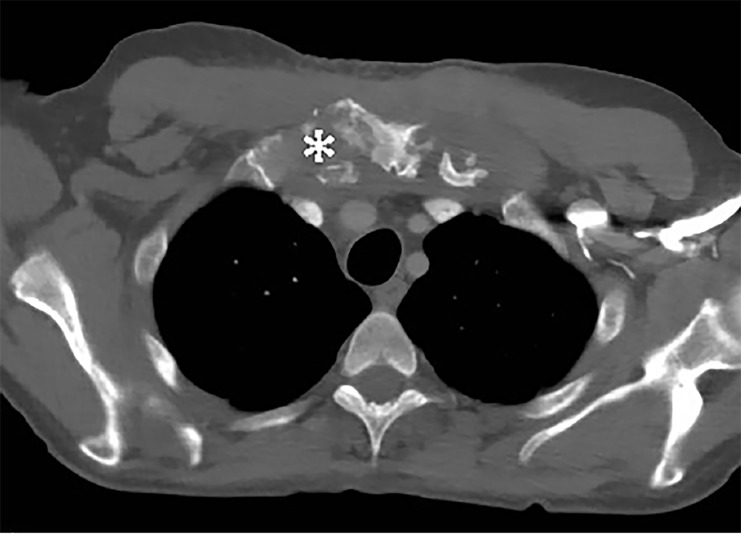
Transverse computed tomography image of the chest, taken at the level of the manubrium, showing widespread destruction of the bony tissue (*).
